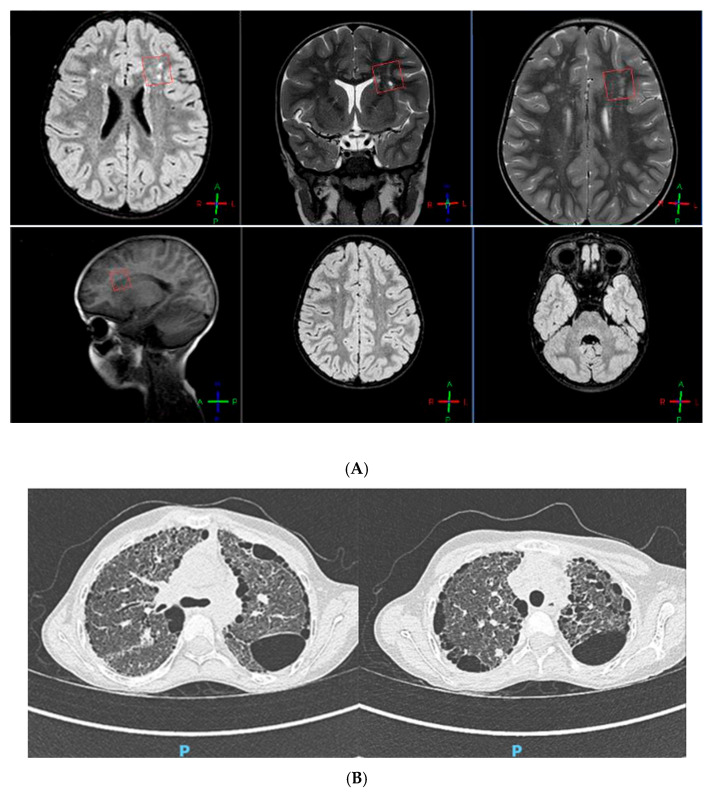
(A) Brain MRI showing leukoencephalopathy. (B) Thoracic CT scan showing “ground glass” and “honeycomb” patterns.
